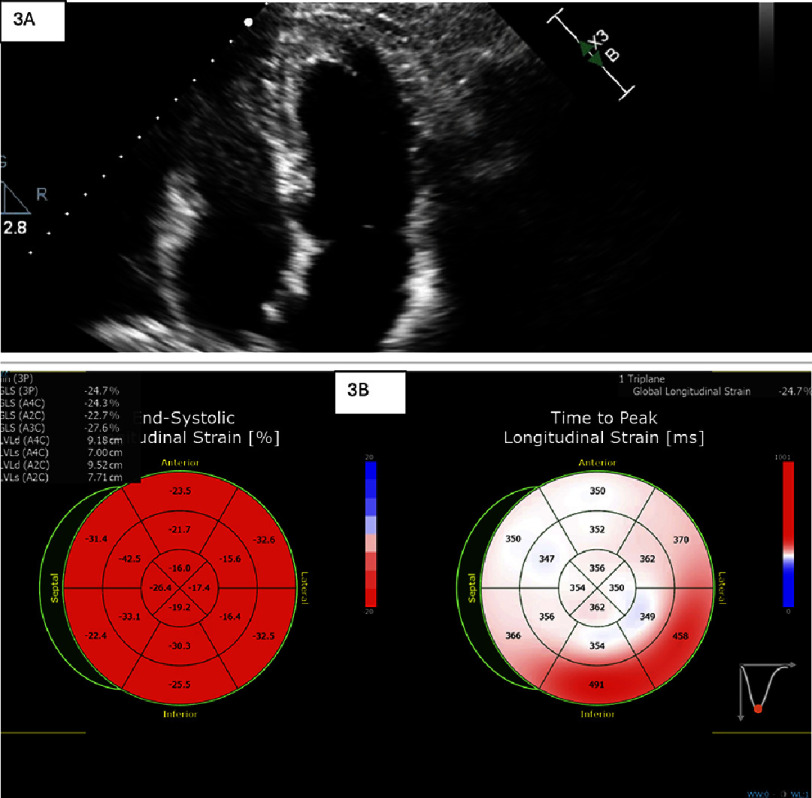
Transthoracic echocardiogram demonstrated normal left ventricular systolic function and chamber dimensions (Figure 3A). Global longitudinal strain was also within normal limits, as shown in Figure 3B.
